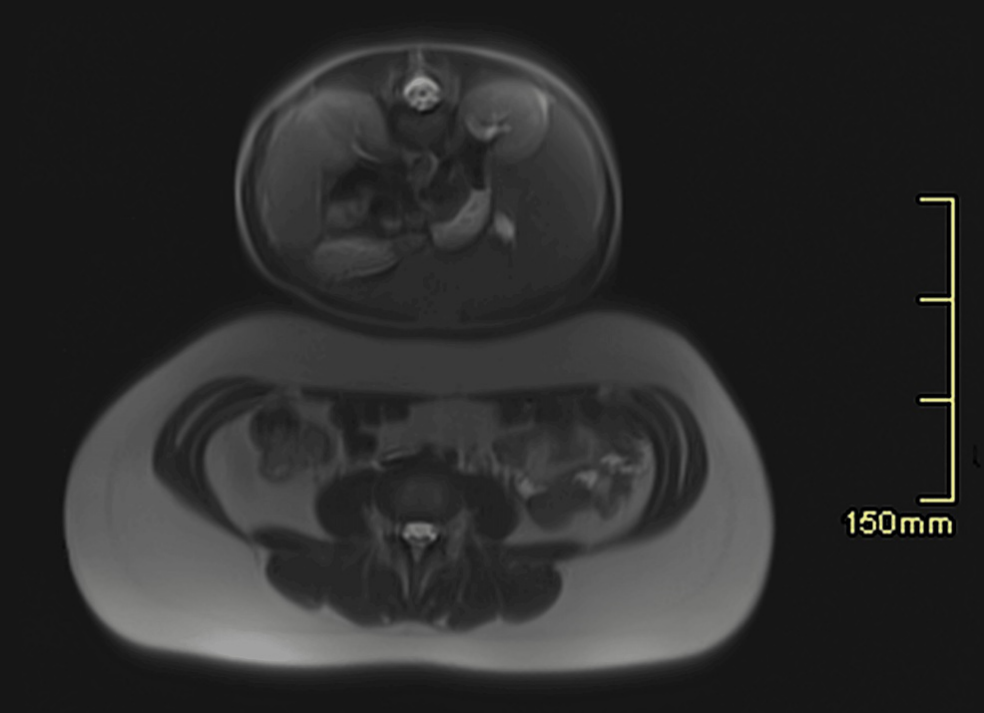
1.5 T MRI axial scout image with a relatively large field of view showing the child atop his mother’s abdomen.
